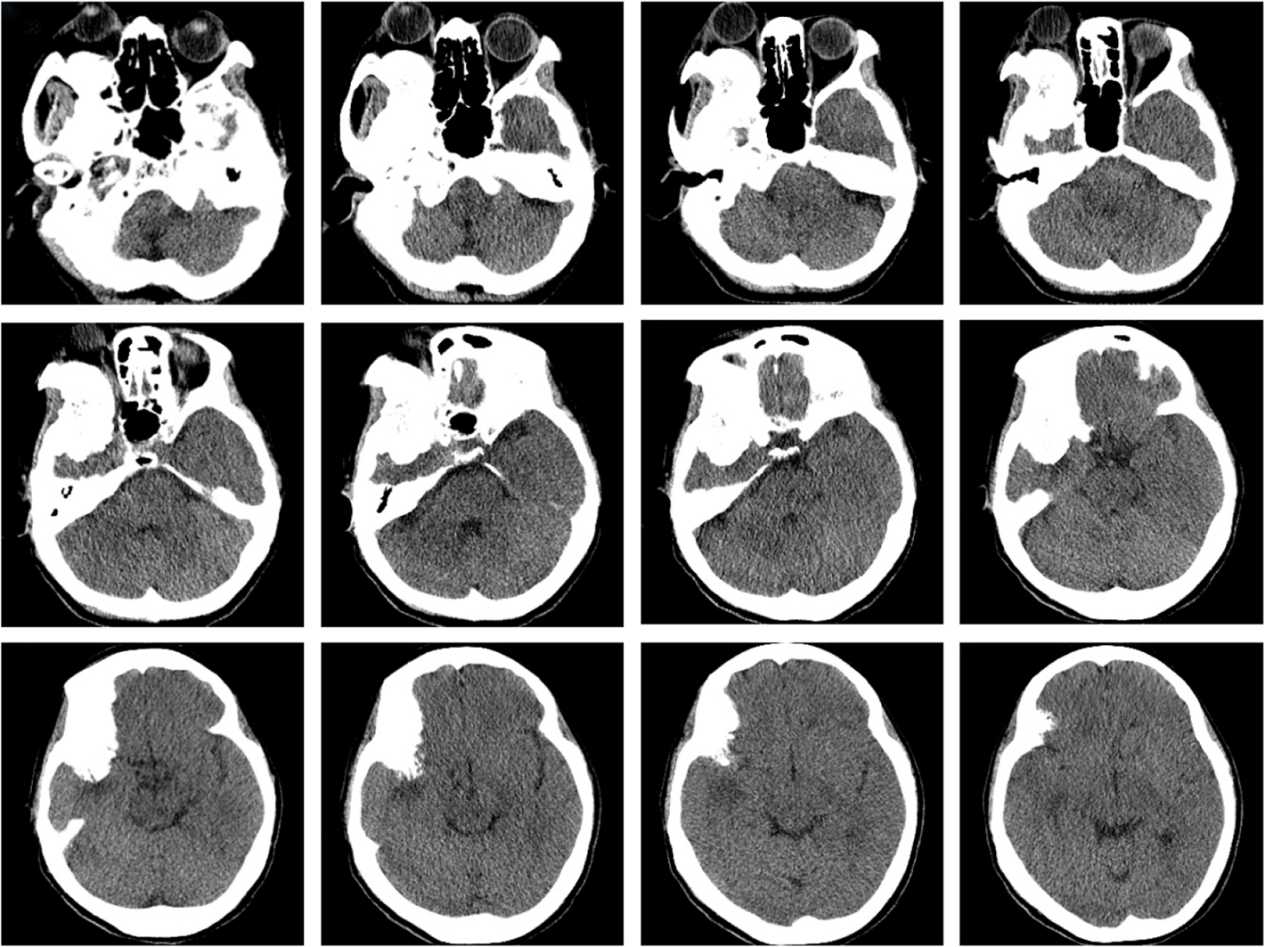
CT scan showing osteolytic honeycomb changes (arrow). The lesion originates from the right wing of the sphenoid bone, extends to the ipsilateral middle cranial fossa and orbit
